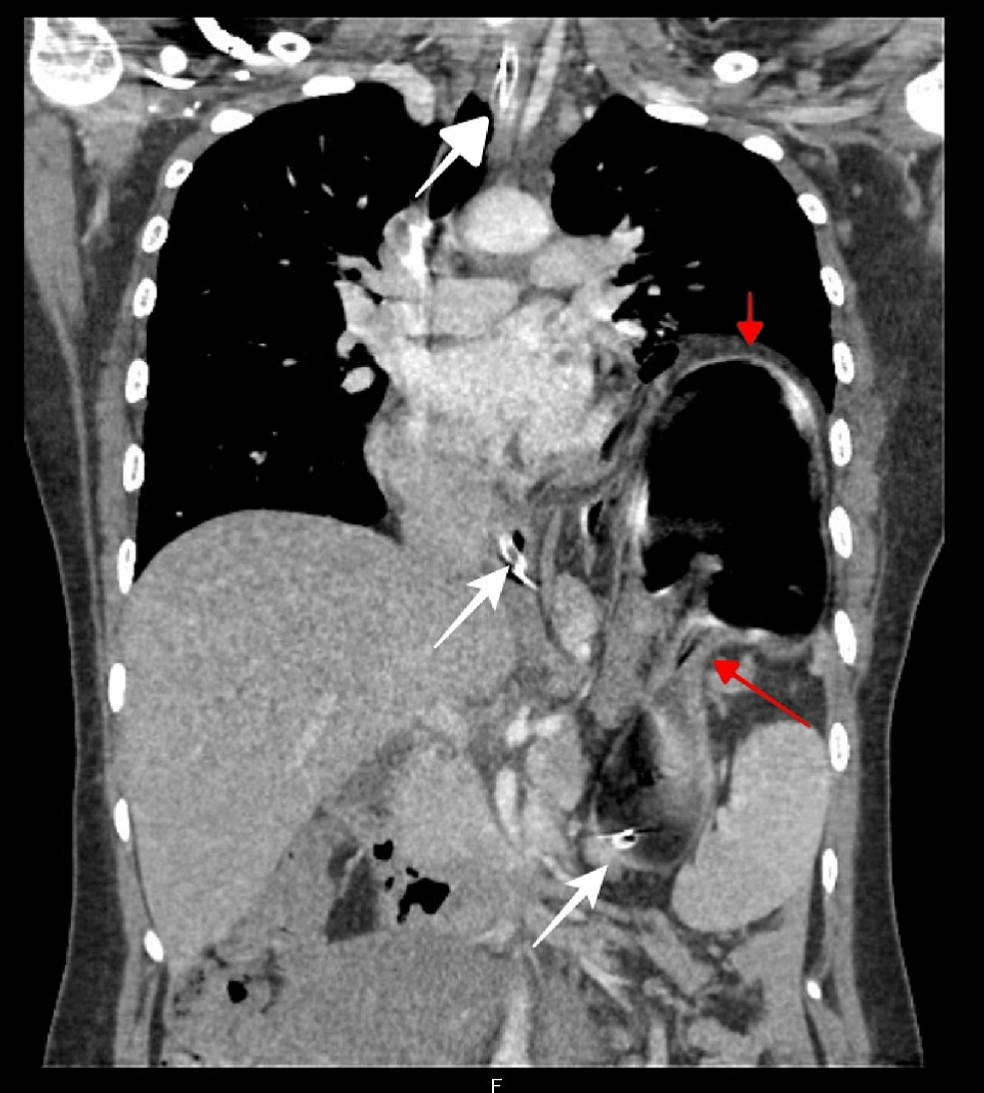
CT thorax and upper abdomen coronal section showing diaphragmatic herniation of stomach with organo-axial volvulus (red arrows) and a nasogastric tube inserted (white arrows). CT, computed tomography
